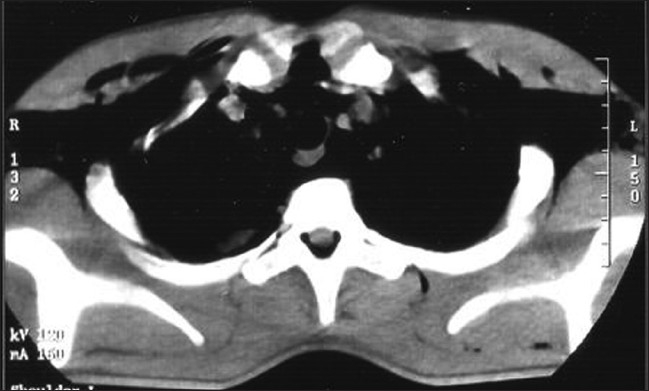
CT chest showing posterior pneumorrhachis
